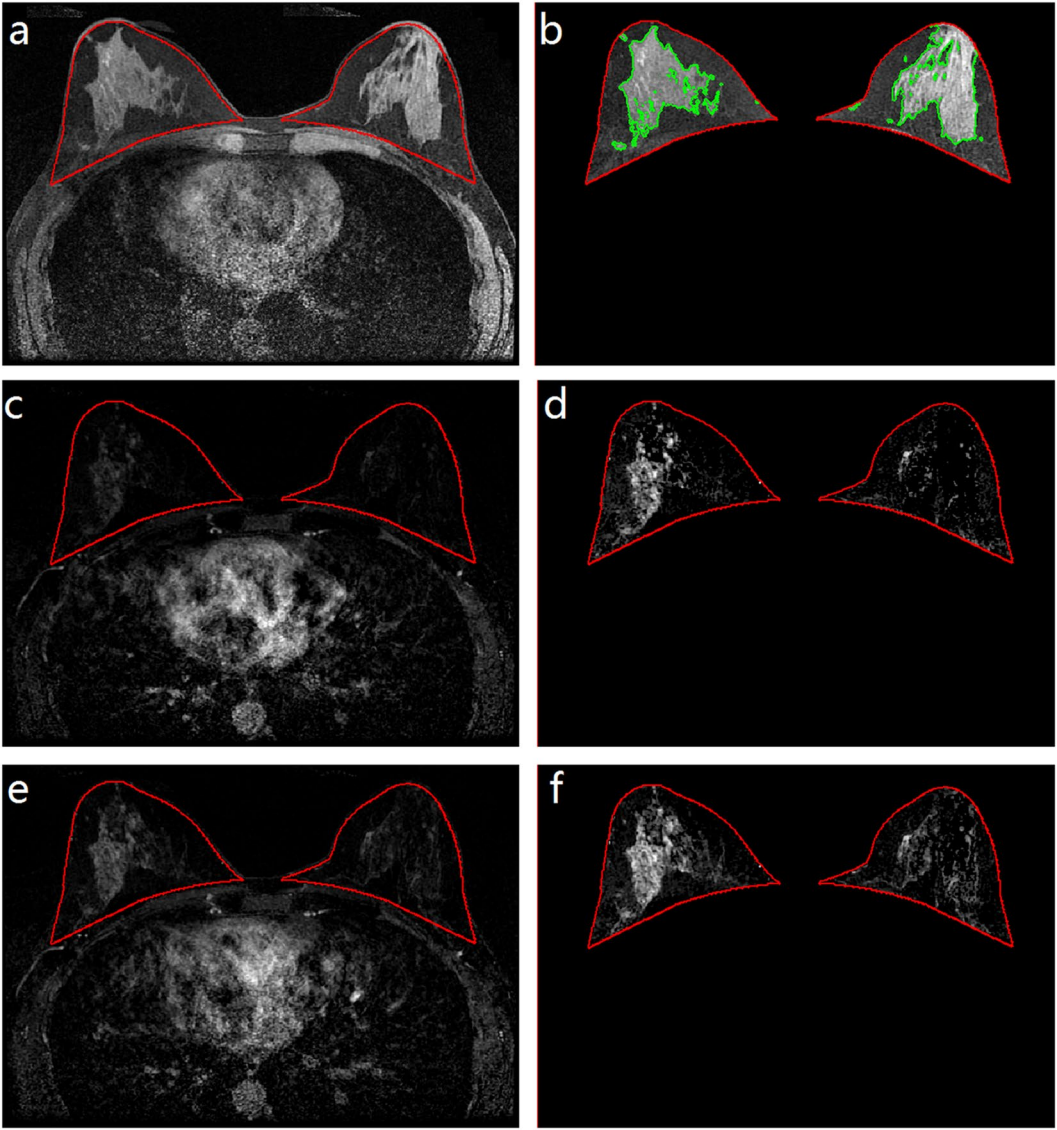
Automated segmentation of background parenchymal enhancement (BPE) and fibroglandular tissue (FGT) from breast DCE-MRI scans. (a) Whole-breast segmentation (red contour) from a pre-contrast image. (b) FGT (outlined by green contour) estimated from the segmented whole-breast. (c) The corresponding first post-contrast-subtracted (SUB1) image with superimposed breast contour (red). (d) BPE estimated from the SUB1 image (plot c). (e) The corresponding third post-contrast-subtracted (SUB3) image with superimposed breast contour (red). (f) BPE estimated from the SUB3 image (plot e).
